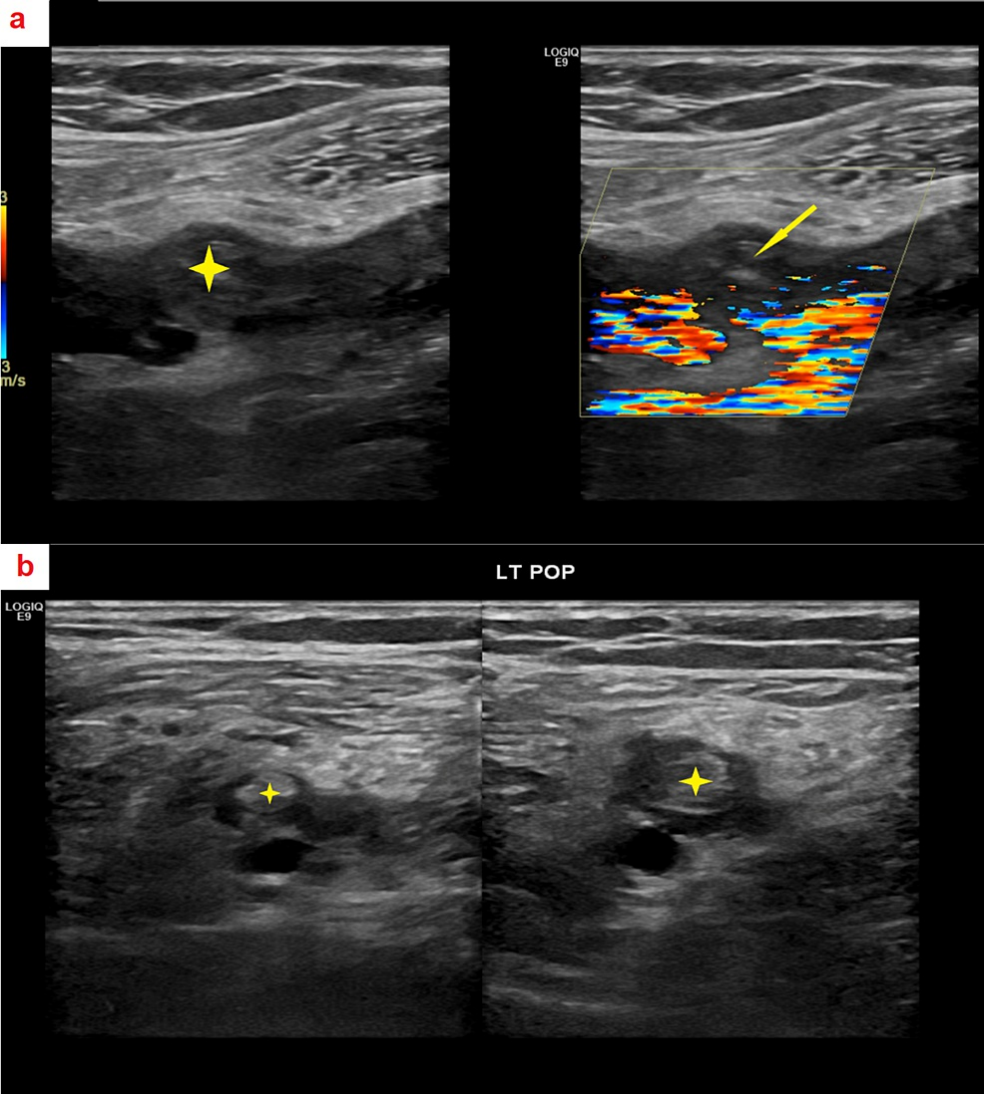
Bilateral lower limb Doppler imaging. a-b: Acute DVT in the left distal superficial femoral, popliteal, and portions of the left and right posterior tibial veins.
